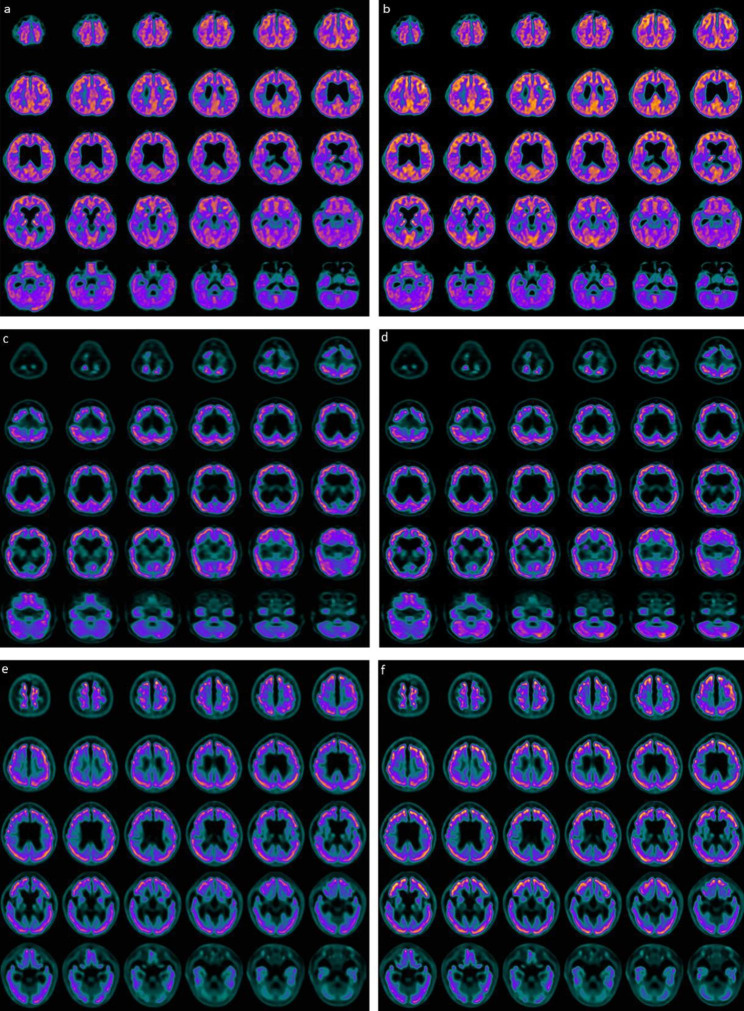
Brain 18 F-FDG PET axial slices before and after combined treatment with hr-NGF and tDCS. (a) In the first patient, before the combined treatment, a moderate global reduction in 18 F-FDG uptake was observed in all cortical regions, while radiotracer uptake was slightly decreased in all subcortical areas. (b) After the combined treatment, an increase in radiotracer uptake was detected in the right and left frontal cortices (right: +11%; left: +12%), right and left parietal cortices (right: +26%; left: +25%), right and left occipital cortices (right: +26%; left: +27%). (c) Brain 18 F-FDG PET axial slices of the second patient, before the combined treatment with hr-NGF and tDCS, showed a severe global reduction in 18 F-FDG uptake in all cortical and subcortical regions, more pronounced in the latter. (d) After the combined treatment, an increase in radiotracer uptake was detected in the caudate nucleus, bilaterally (right: +51%; left: +51%) and cerebellum (+ 20%). (e) In the third patient, brain 18 F-FDG PET axial slices before the combined treatment showed a mild global reduction in 18 F-FDG uptake in all cortical regions and a more severe reduction in all subcortical regions. (f) After the combined treatment, an increase in radiotracer uptake was detected in the bilateral frontal cortex (right: +29%; left: +34%), bilateral parietal cortex (right: +15%; left: +19%), right and left occipital cortices (right: + 20%; left: +19%) and cerebellum (+ 14%)
